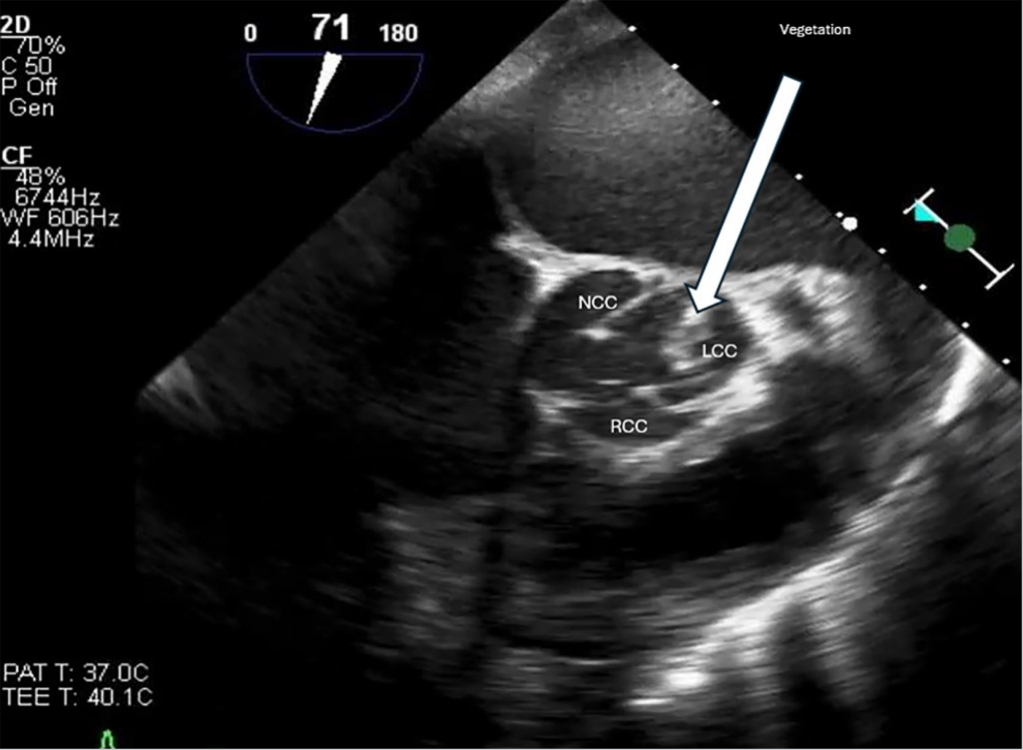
Transesophageal echocardiography (TEE) on day 10 post-readmission to medical intensive care unit (MICU) with mid esophageal short axis view showing vegetation on the left coronary cusp (LCC). LCC, left coronary cusp; RCC, right coronary cusp; NCC, non-coronary cusp.
